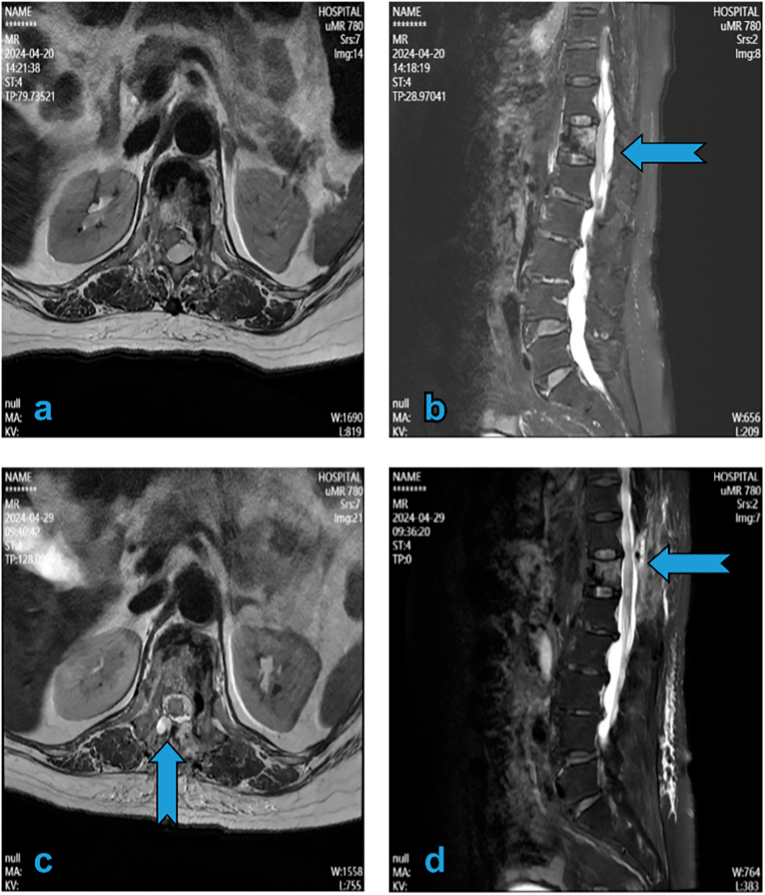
T2 vertebra MRI scan. (a and b) Hyper-acute epidural hematoma over T11 extending to the L1 vertebrae. Axial images showing epidural hematoma compressing the spinal cord (arrow). (c and d) MRI after hemilaminectomy decompression and hematoma removal showing resolution of both SEH and nerve compression (arrow). MRI: magnetic resonance imaging; SEH: spinal epidural haematoma.
